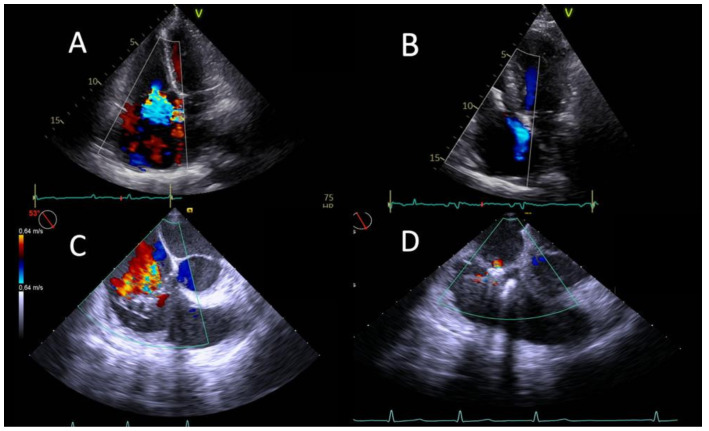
Echocardiographic pictures of successful TR reduction after TEER. Transthoracic echocardiographic four-chamber view at baseline (A) and at discharge (B) after implantation of one TriClip XT. Transoesophageal echocardiographic right ventricle inflow/outflow view showing severe tricuspid regurgitation mainly originating from septal and anterior leaflet at baseline (C) and traces of regurgitation after implantation of one device (D).
